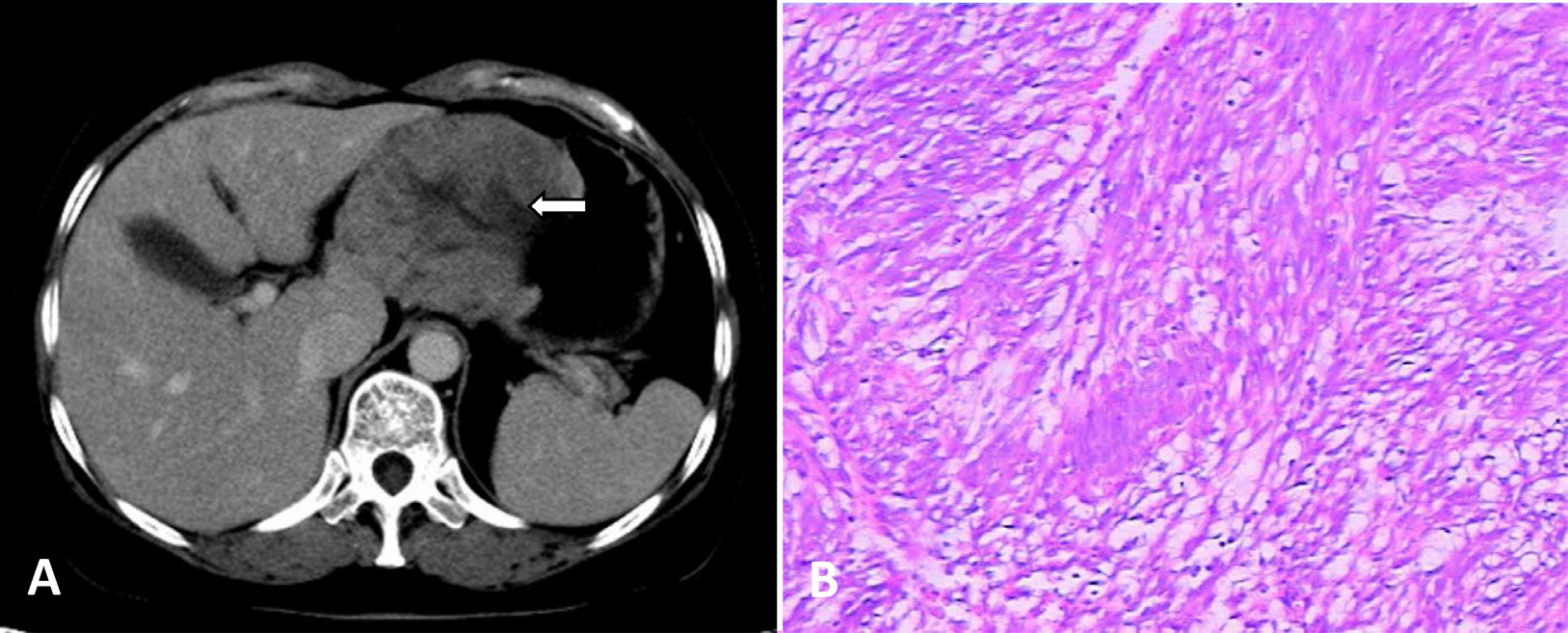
Computed tomography (CT). CT obtained on May 19, 2015, before surgery for a gastric stromal tumor revealed a gastric mass (A);Postoperative pathology revealed a high-risk “gastric” stromal tumor (B).
